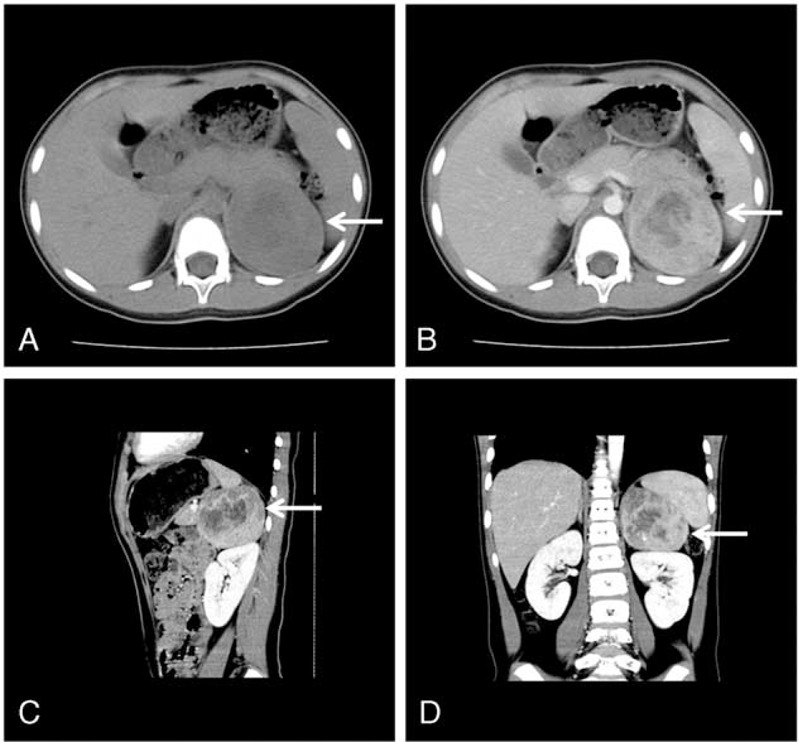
CT scan showing an ovoid tumor (arrow) with mixed signal intensity, calcification, and inhomogenous enhancement. Axial view (A) and intravenous contrast-enhanced axial (B), sagittal (C), and coronal (D) views. CT = computed tomography.
